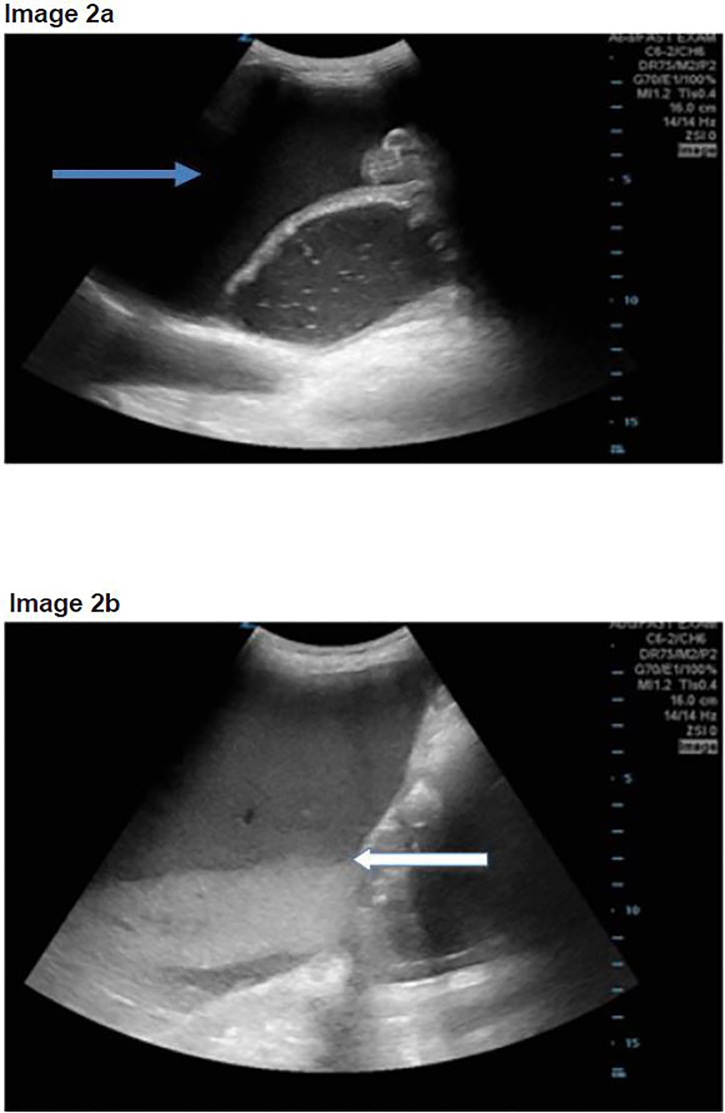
a) Point-of-care ultrasound (POCUS) with dilated stomach and surrounding intraperitoneal free fluid (blue arrow); b) POCUS with “black-and-white cookie” sign seen by white arrow with division of stomach contents.
